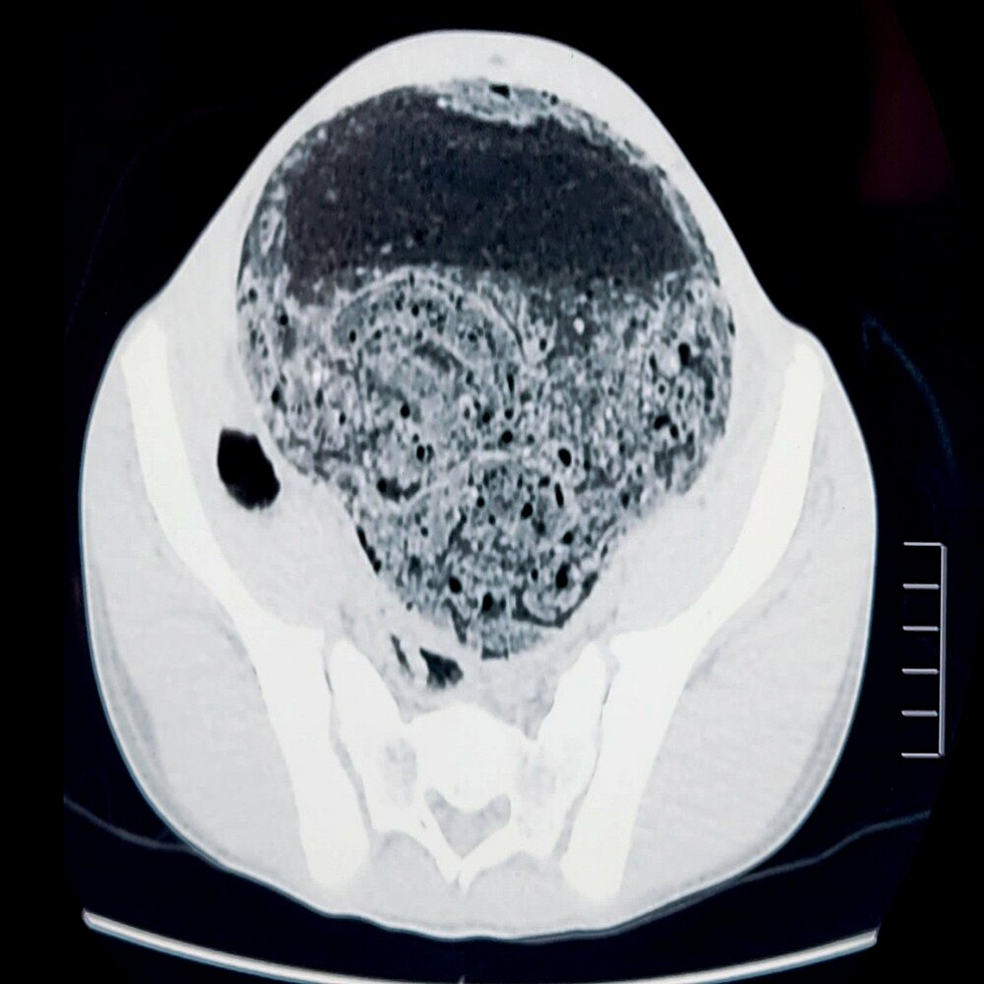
CT scan showing dilated colon in the axial section.
